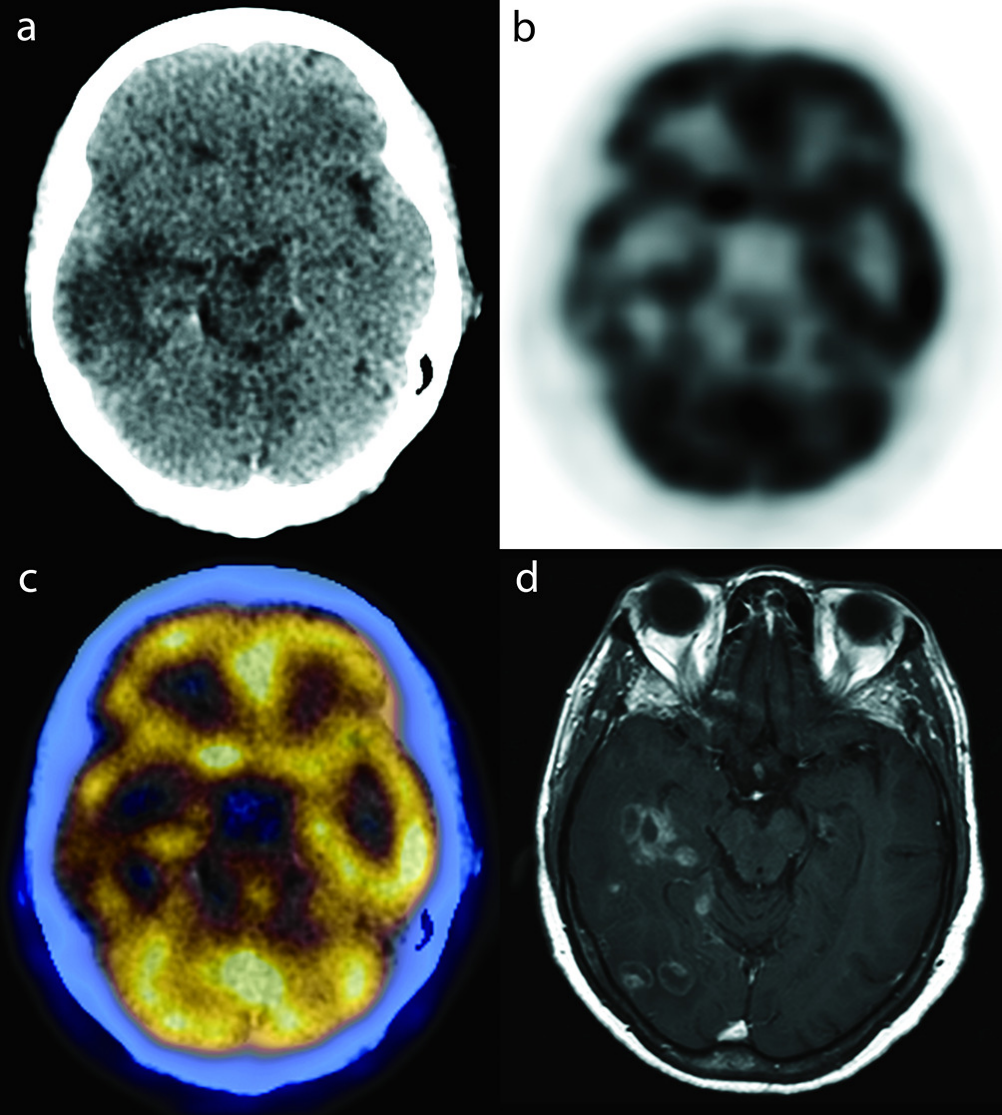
(a) Transaxial CT, (b) PET, (c) PET/CT fusion and (d) MRI *T*_1_ weighted post-gadolinium images. The intensely enhancing cerebral lesions seen on MRI appear hypometabolic on the PET/CT images when compared with normal grey matter. PET, positron emission tomography.
